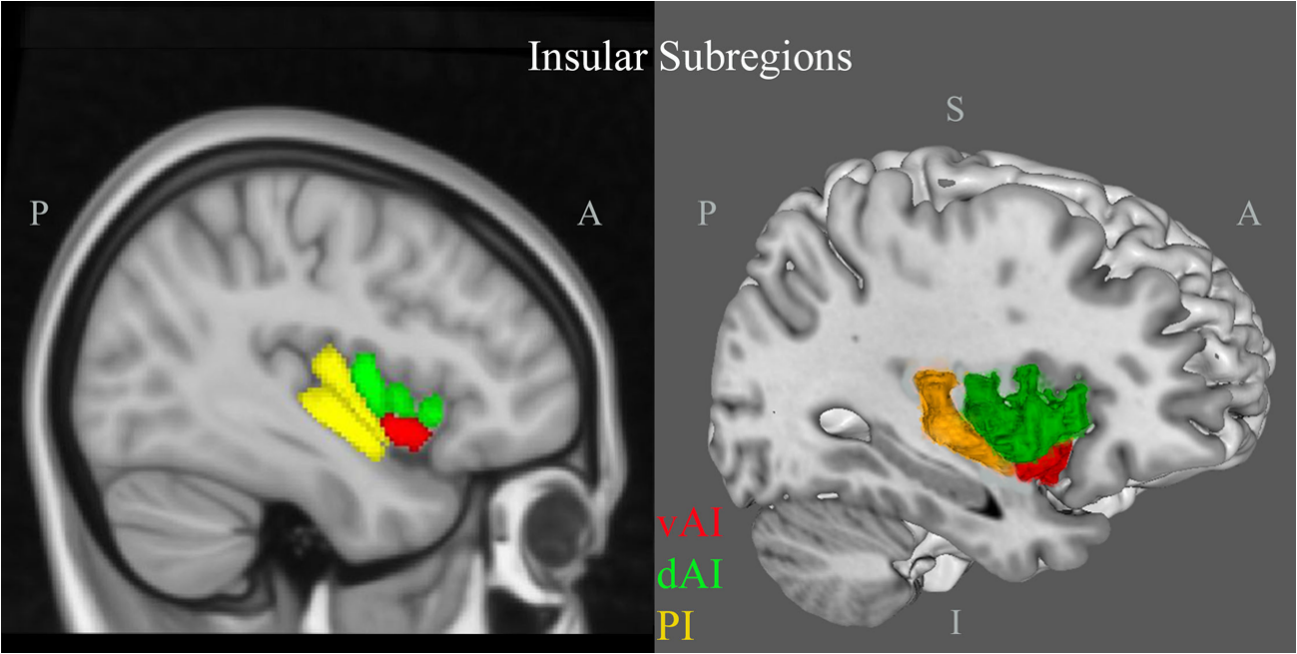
Insular Cortex Sub-regions in MRI. The central sulcus generally divides the insular cortex into anterior and posterior subdivisions. The anterior insular cortex is further divided into ventral (red) and dorsal sub-regions (green) and the dorsal insula can be divided into an anterior and mid-region. The left figure shows the sub-regions of the insular cortex based on the Hammers_mith probabilistic atlas (Faillenot et al., 2017). Surface rendering of the insular sub-regions in 3D is shown on the right. A: anterior, dAI: dorsal anterior insula, I: inferior, P: posterior, PI: posterior insula, S: superior, vAI: ventral anterior insula
